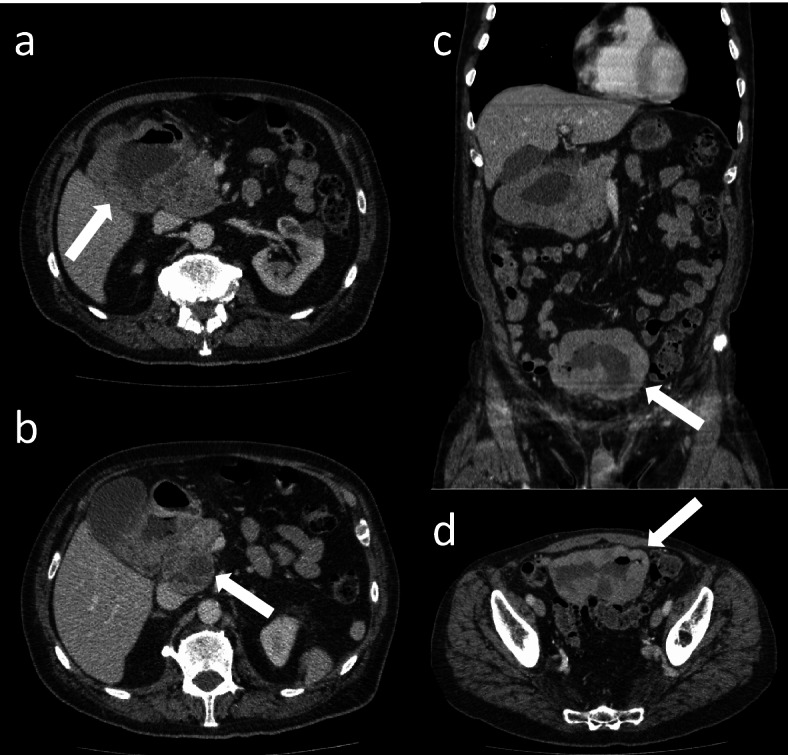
a and b Axial CT images of the abdomen with a large cavitated aneurysmal mass in the duodenum (arrow in a) with an adjacent necrotic node (arrow in b). c coronal image showing the aneurysmal dilatation of the duodenum and ileum (white arrow), the latter also present in d in the axial plane
